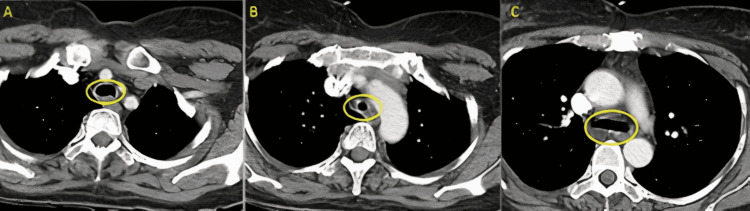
CT Angiogram Pulmonary Embolism with Omnipaque Impression: There is abnormal narrowing and associated abnormal thickening of the wall of the distal trachea. The differential diagnosis includes both inflammatory and neoplastic etiologies. Pulmonary consultation and bronchoscopy is recommended for further evaluation of the trachea. A) Axial view, superior to the level of tracheal stenosis; B) Axial view, at the level of the stenosis; C) Axial view, at the level of the carina and below the level of tracheal stenosis.
